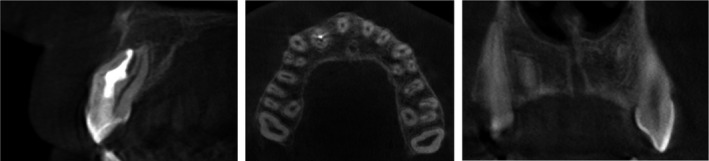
Cone‐beam computed tomographic (CBCT) imaging in 12‐month follow‐up shows successful healing of bone structures
